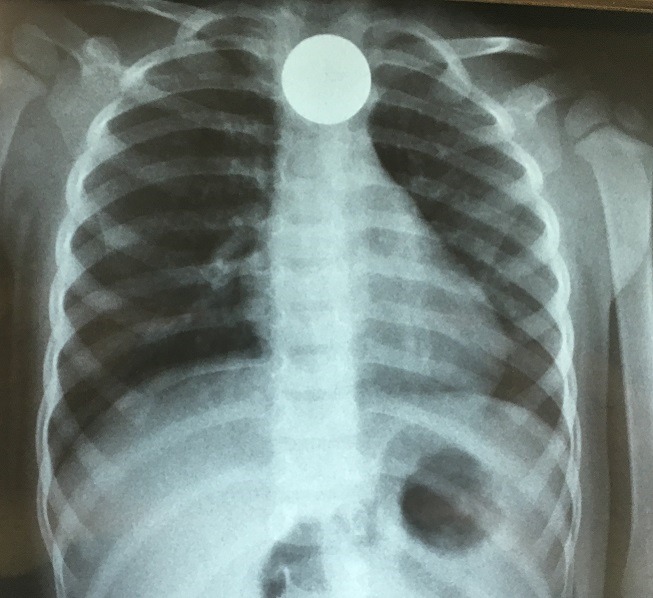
Radiographie pulmonaire de face montrant une pièce de 25 francs CFA bloquée dans le tiers supérieur de l’œsophage
